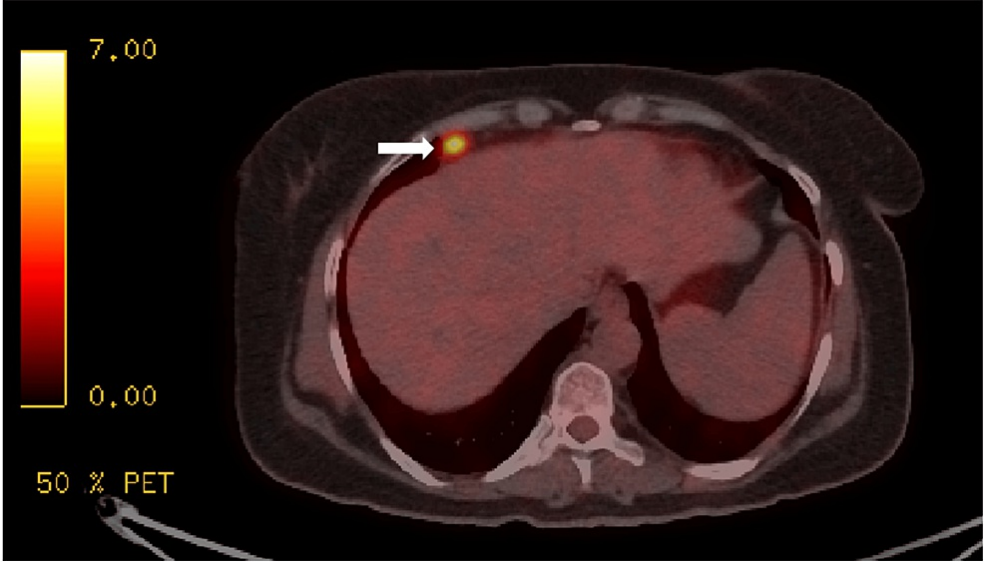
Positron emission tomography/CT revealed new lymphadenopathy in the chest (right sub-diaphragmatic new lymph node visually indicated by a white arrow).
